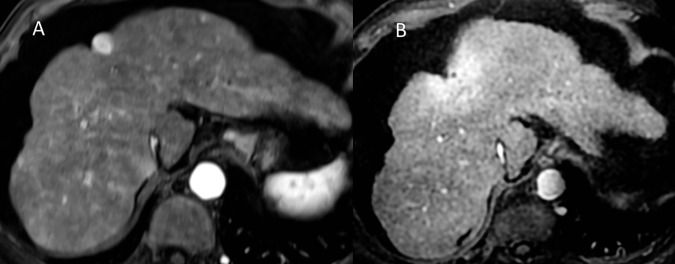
A 52-year-old man with HCC. 5A. Sub-capsular HCC on arterial phase on dynamic 3D T1 weighted imaging at the time of diagnosis. 5B. MRI at six months: portal phase on dynamic 3D T1 weighted imaging. Post-treatment changes with hypervascular ring and capsular retraction.
